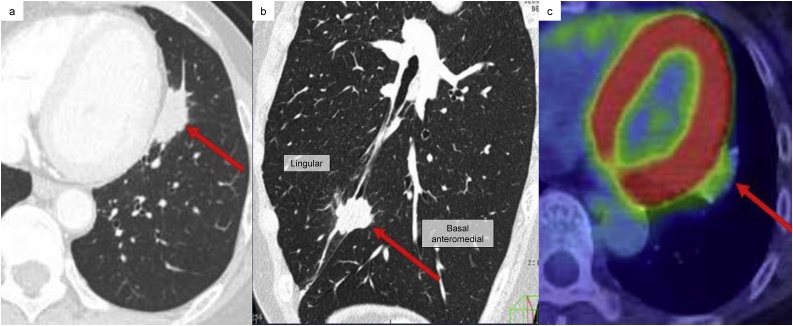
Radiological imaging findings. a, b) Computed tomography (CT) shows a nodule located between the lingular and basal anteromedial segments in the incomplete lobulation. (a; Axial, b; Sagittal image). c) Positron emission tomography (PET)-CT shows abnormal uptake in the tumour with standardized uptake value max of 2.8. The red arrow indicates the tumour.
